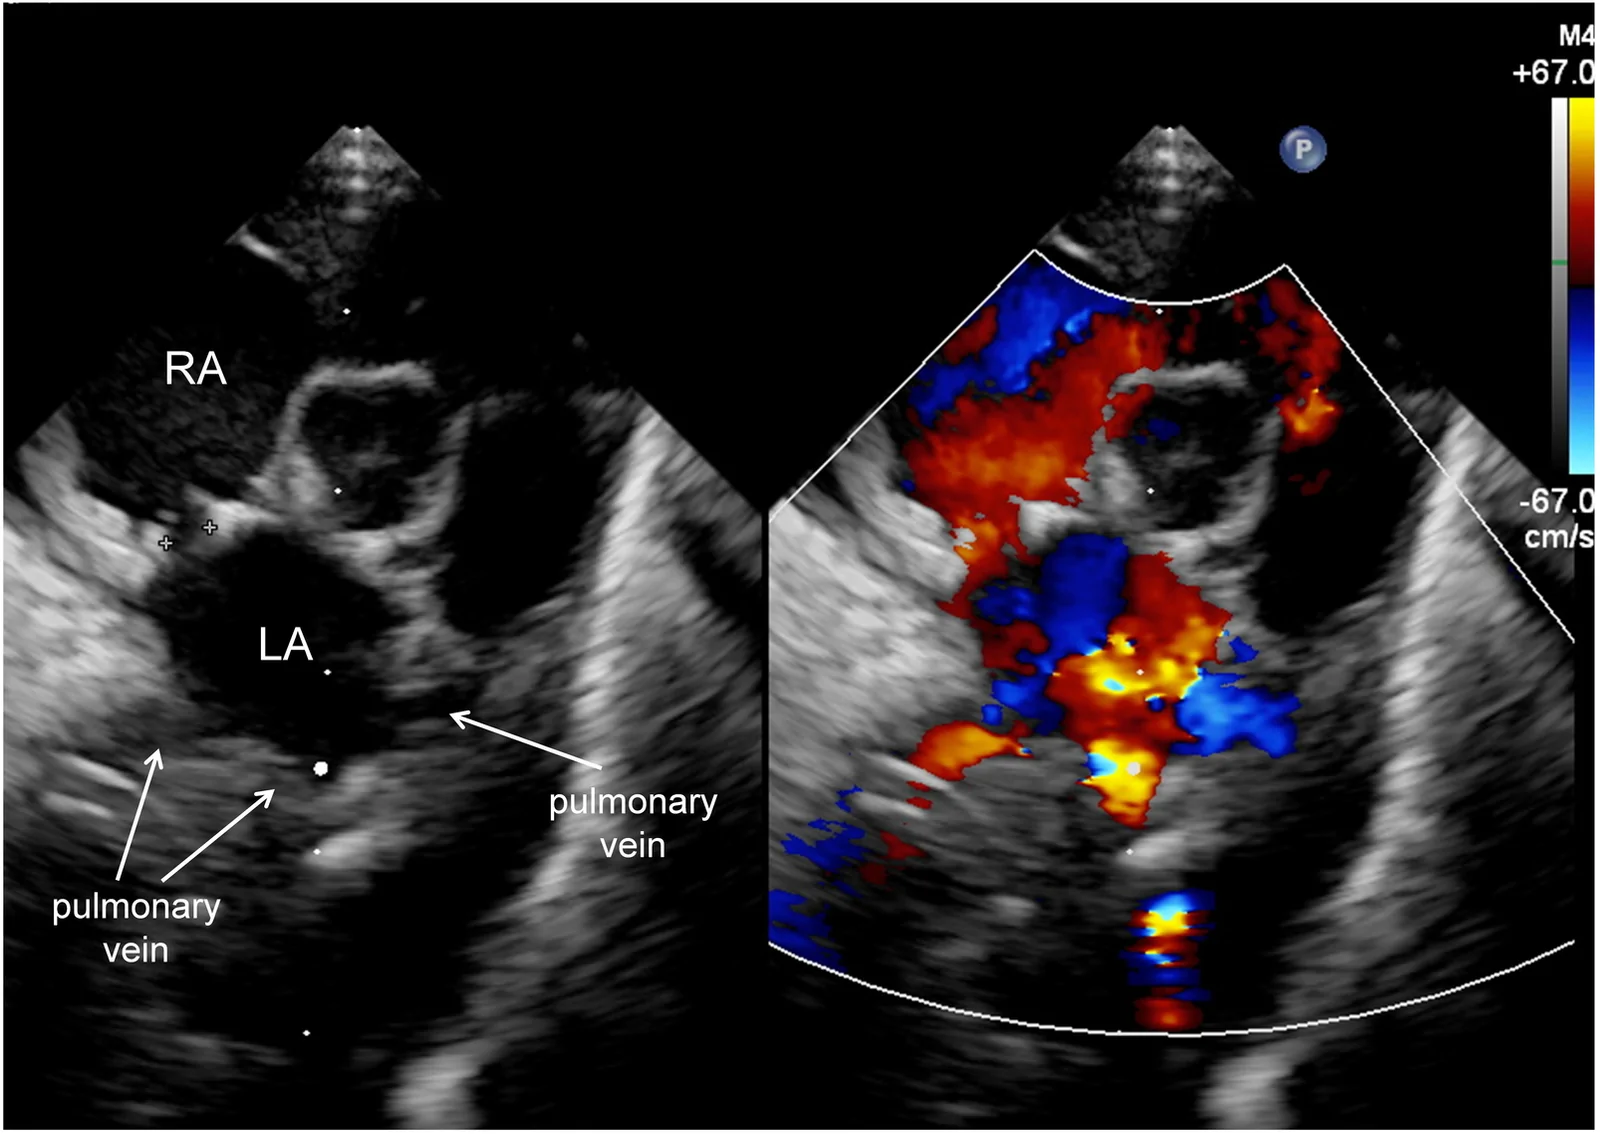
Two-dimensional echocardiography in the immediate postoperative period after sutureless repair of CPVA shows unobstructed pulmonary venous drainage into the left atrium. *CPVA*, common pulmonary vein atresia; *RA*, right atrium; *LA*, left atrium.
